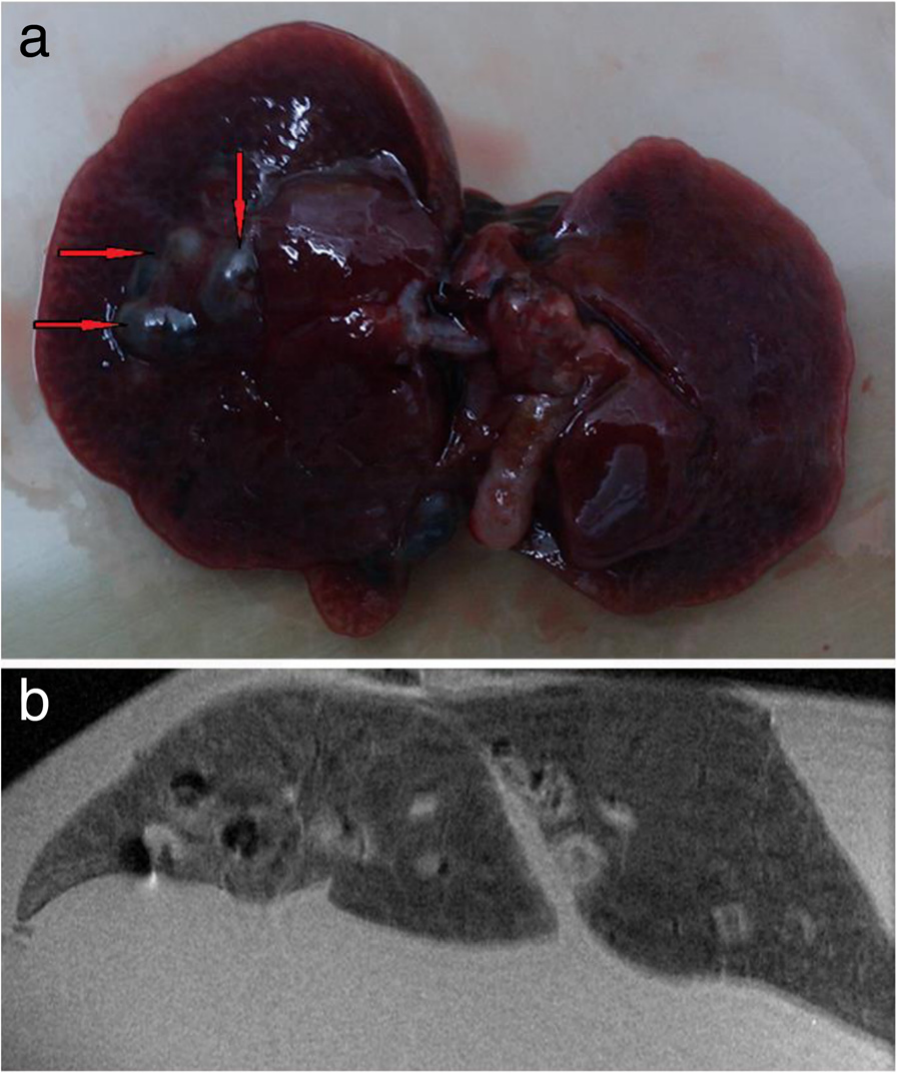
Detection of hemozoin knobs by magnetic resonance imaging. (a) The macrophotograph of *Opisthorchis felineus* infected hamster liver at 12 week postinfection. The hemozoin knobs in bile duct are marked by pointer; (b) *ex vivo* MRI of this liver in PBS buffer. The hemozoin knobs are visualised as dark areas
